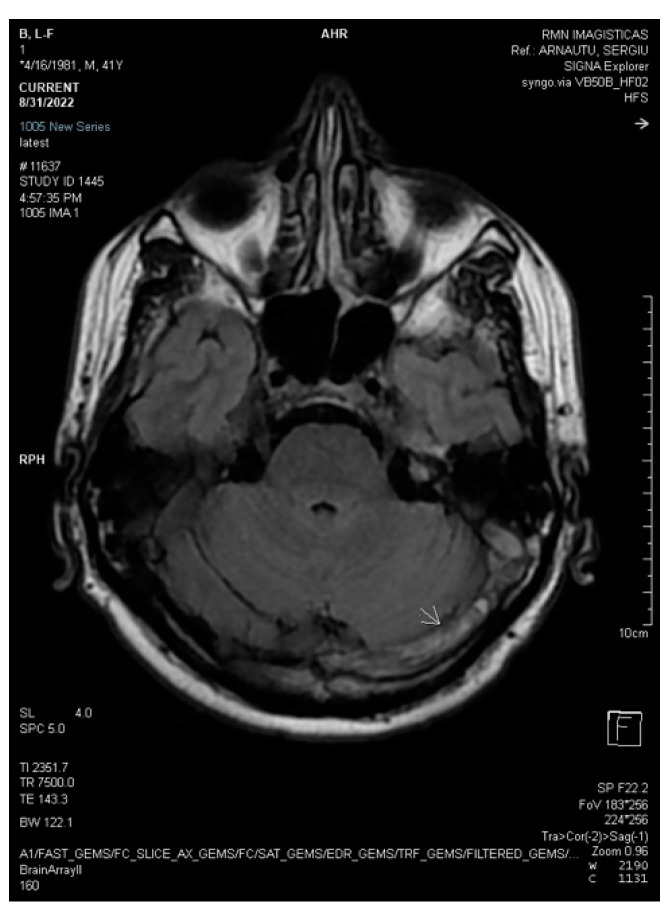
2D axial FLAIR shows reduced flow in the left transverse sinus (white arrow).
